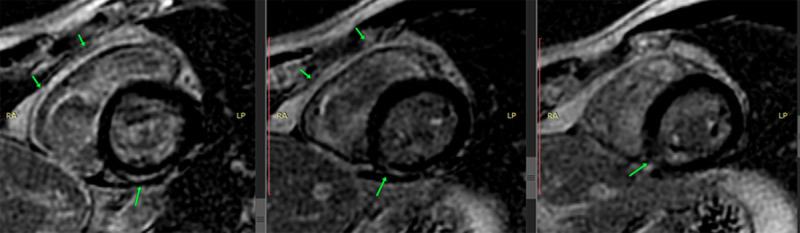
Cardiac MRI with late gadolinium enhancement in the inferoseptal, inferior, and inferomedial segments.
